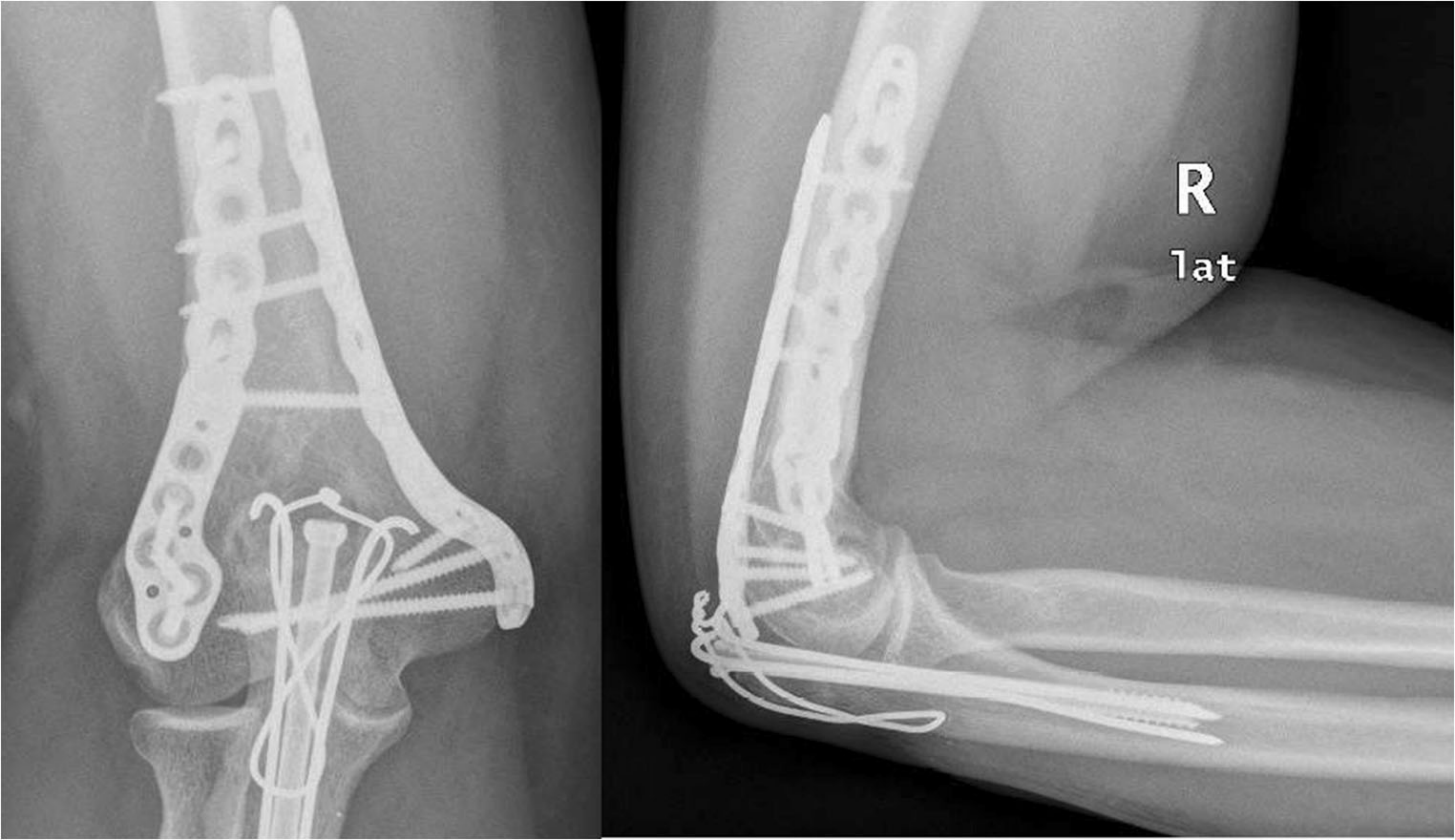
Anteroposterior and lateral elbow radiographic views of 90°–90° orthogonal plating of a distal humerus fracture
